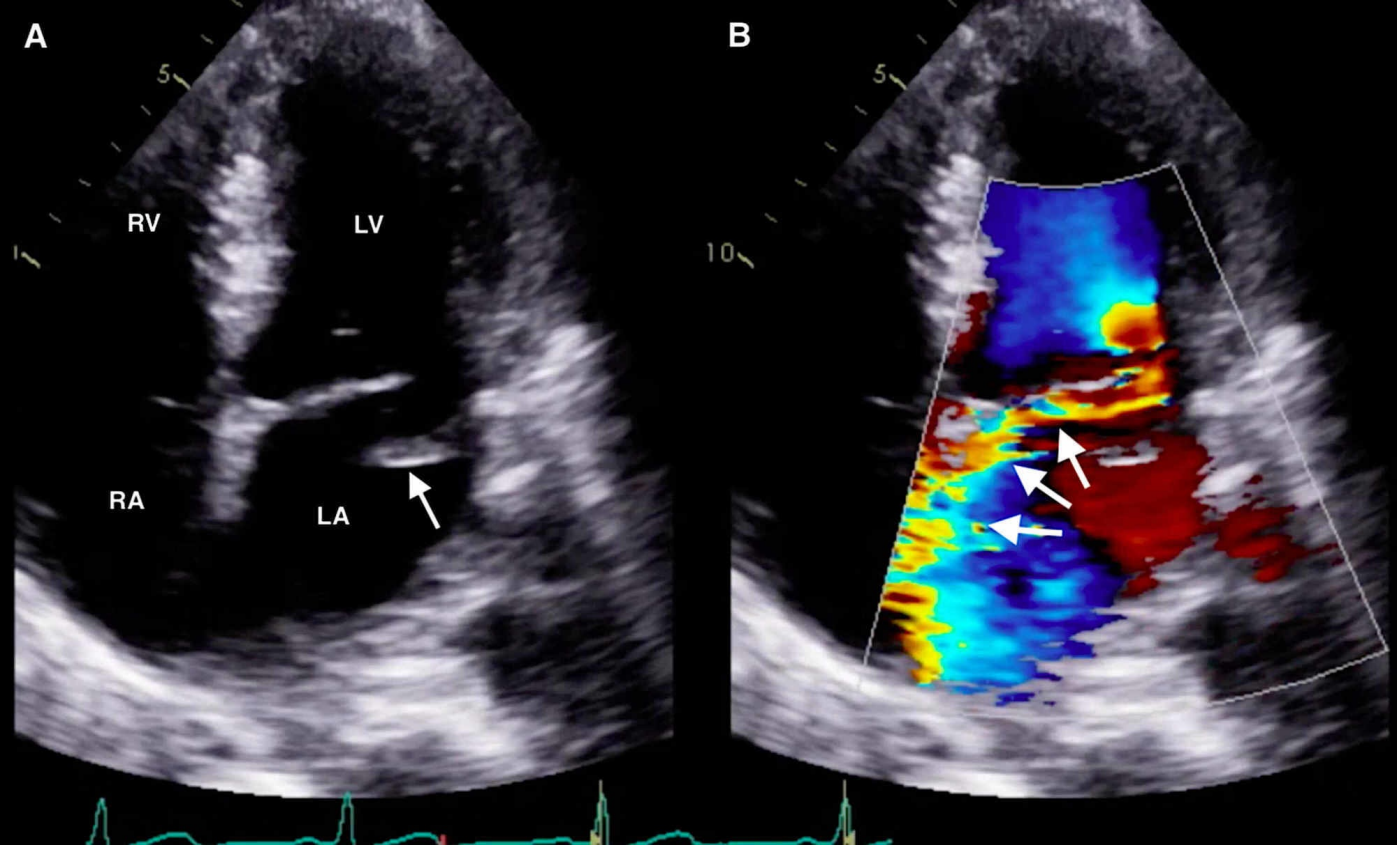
Comprehensive transthoracic echocardiogram (apical four-chamber window) demonstrating (A) flail motion of the posterior mitral leaflet and (B) severe eccentric mitral regurgitant jet (arrows). RA, right atrium; RV, right ventricle; LA, left atrium; LV, left ventricle.
